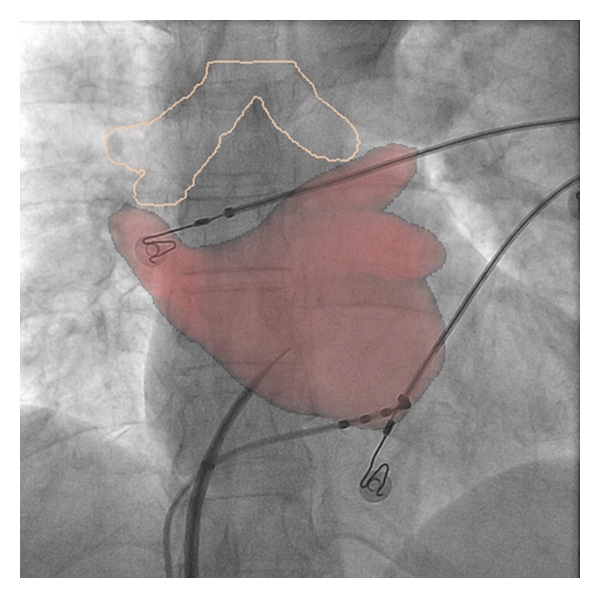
Real-time respiratory-gated motion compensation. A system is being developed whereby the 3D shell of the left atrium (either from CT/MR or rotational angiography) moves during the abaltion in response to respiratory motion. This example demonstrates how the trachea is automatically tracked. Image courtesy of Kawal Rhode, Kings College London and St. Thomas' Hospital.
